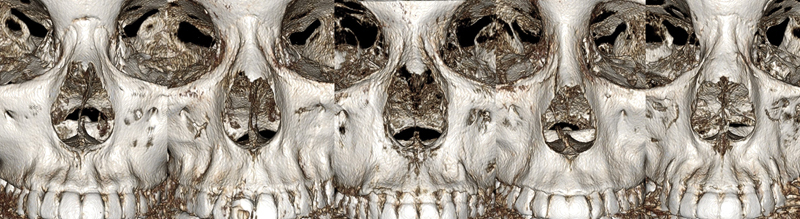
Various features of nasal bone and pyriform aperture in different individuals on 3D CT scan.
